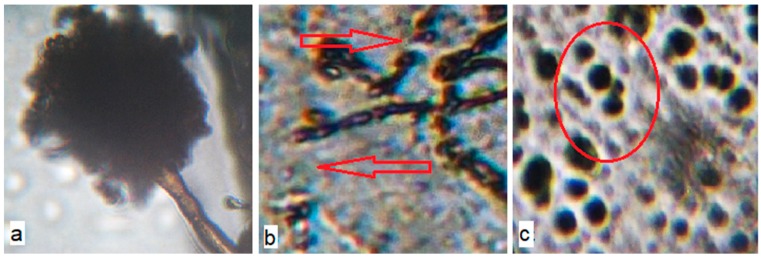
Microphotographs of the surface of the inoculated polyethylene (PE) materials with birch bark extract (BBE) and with the use of ultrasonic treatment on the 14th day of the exposure of *A. niger* (400× magnification): (a) PE 0% BBE, ultrasound; (b) PE 0.5% BBE, ultrasound; (c) PE 2.0% BBE, ultrasound.
